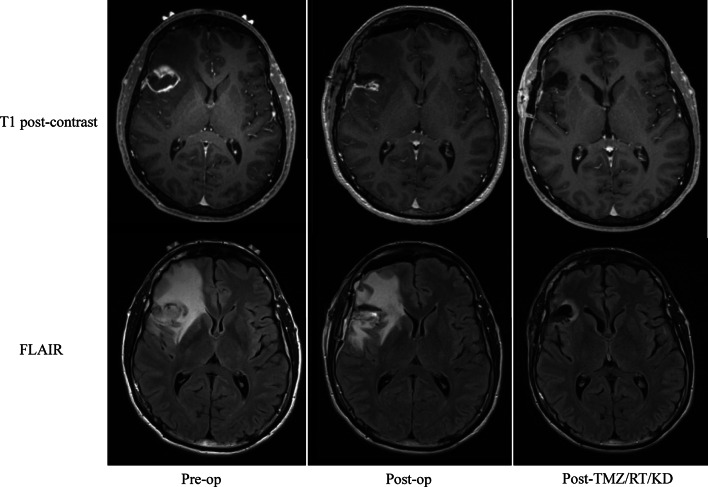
Representative MRI scan before surgery, after surgery, and after RT/chemotherapy/KD initiation. Decreased contrast enhancement and T2/FLAIR hyperintensity is noted on the post-treatment MRI.
